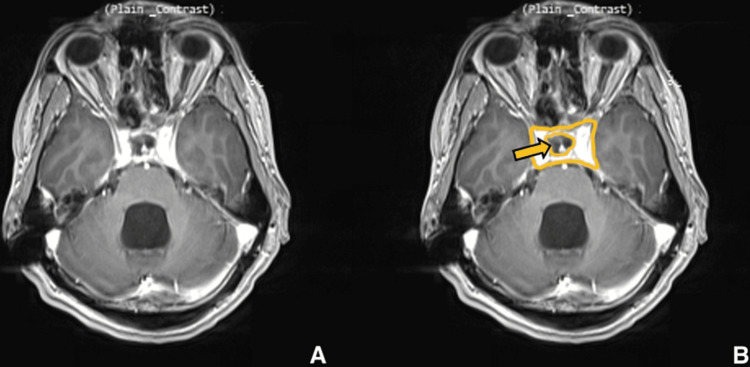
Postoperative imaging for patient 1. Contrast-enhanced T1-weighted axial image showing significant reduction in size of lesion. (A) Without ROI and (B) with ROI. The yellow arrow is pointing to the hypointense region where the mass has been reduced.
